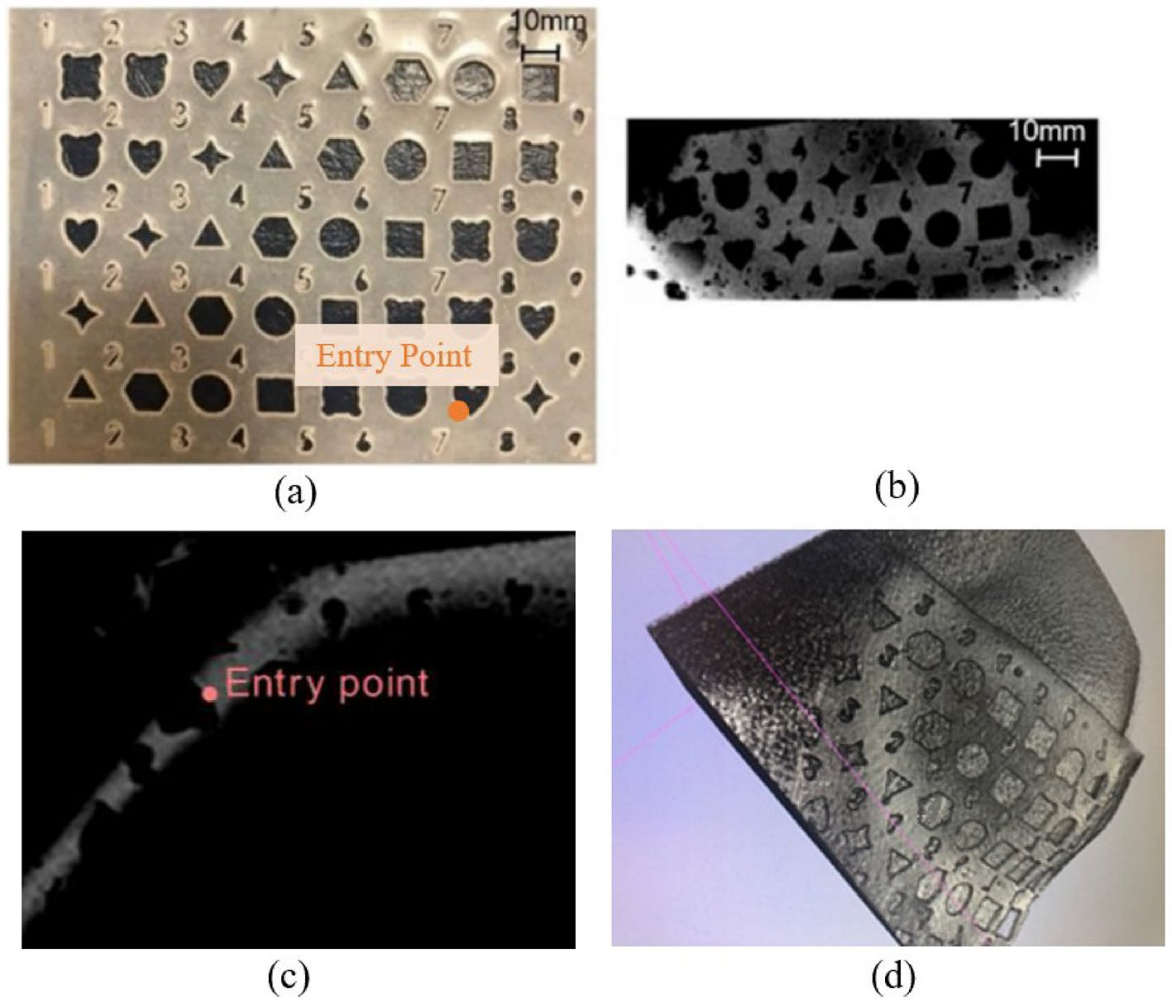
(a) Silicone grid template with a spatially unique, non-repeating pattern, (b) T1-weighted MR images of the silicone grid template, (c) MR image of an out-of-plane grid and a hypothetical entry point, (d) 3D rendered image of the silicone grid made using segmentation of the MRI dataset.
